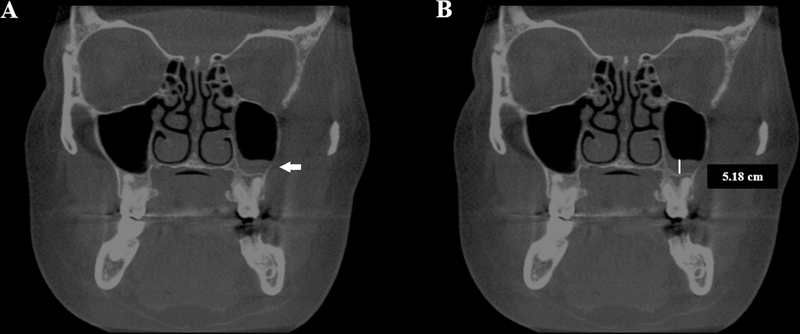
Coronal reconstructions of a CBCT exam showing the mucosal thickening of a maxillary sinus. (
